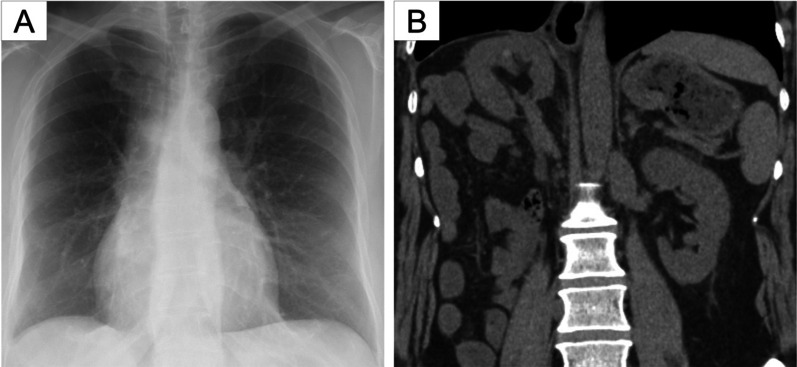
Postoperative chest radiograph (a) and coronal abdominal computed tomographic (CT) scan (b). a Massive area of opacity had completely disappeared. b No recurrence of the diaphragmatic hernia was confirmed 20 months after the operation
